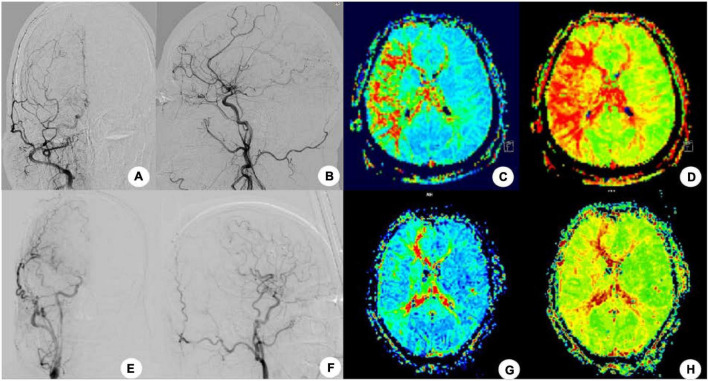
After right superficial temporal artery-middle cerebral artery (STA-MCA) bypass grafting in one patient, no recurrence of stroke and transient ischemic attack was found at 4-month follow-up, and cerebral angiography showed that the bypass supplied blood to the brain. (A,B) Cerebral angiography before the bypass surgery was shown. (C,D) Mean transit time (C) and TTP (D) were shown before surgery in MRI-PWI. (E,F) Cerebral angiography after surgery. (G,H) Four months after surgery, the MTT (G) and TTP (H) were significantly improved.
